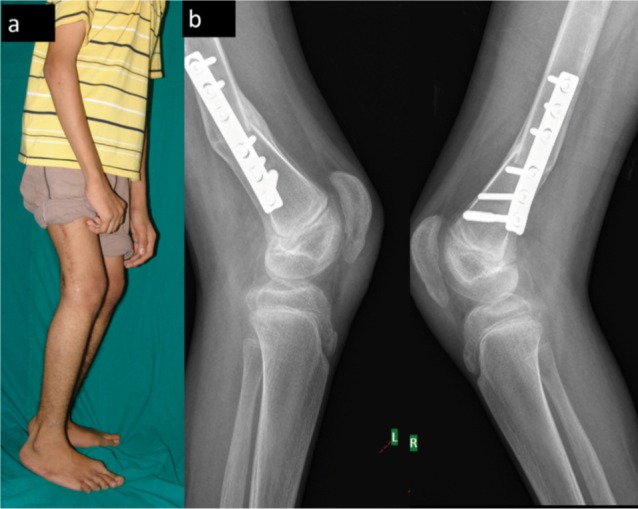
(a) Post-op standing photograph showing significant improvement of gait, and (b) Post-op radiograph of knee showing the correction fixed flexion deformity and patella alta with healing of stress fracture of the patella 1 year following surgery
